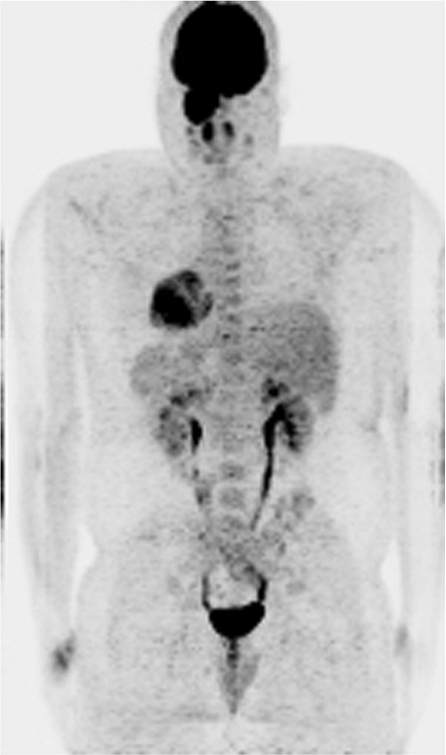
Whole Body PET/CT (a) Diagnostic Staging PET/CT – There is a hypermetabolic mass centered in the left maxillary sinus. The left cervical lymph nodes between the sternocleomastoid muscle and internal jugular vein as well as subcentimeter level 2 lymph nodes are also hypermetabolic.
